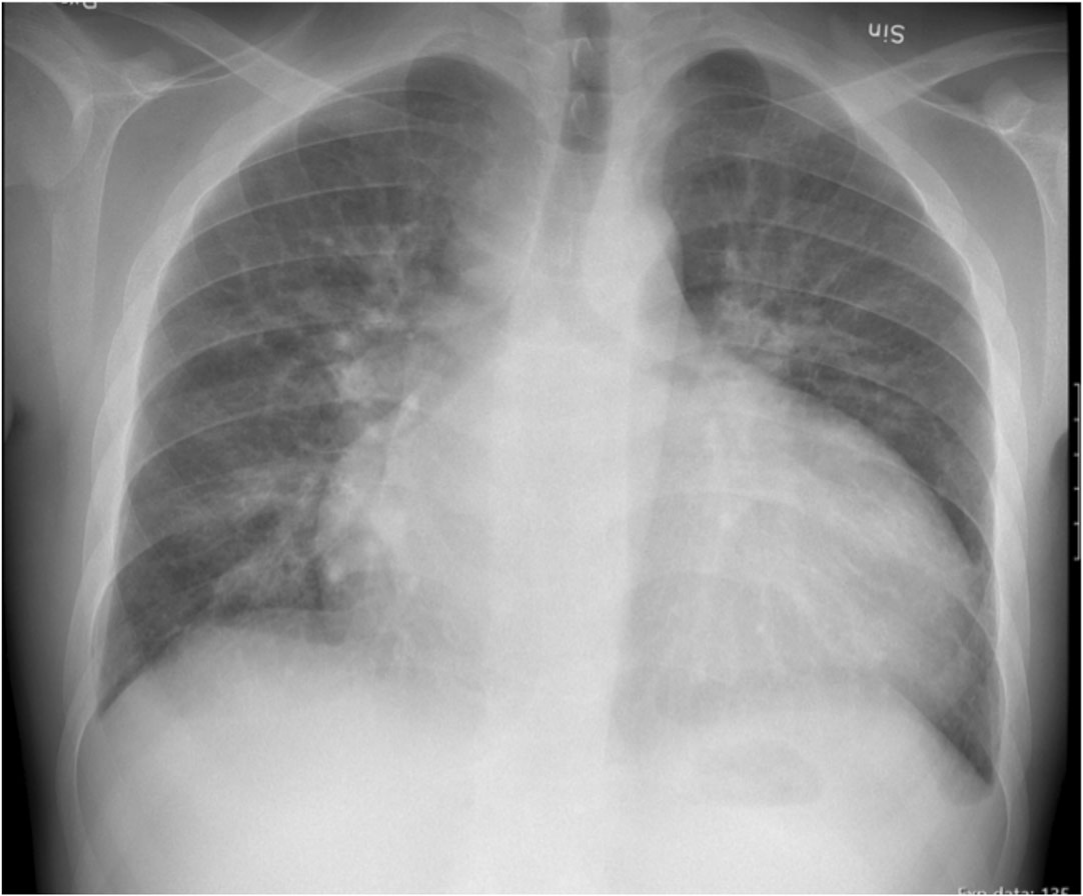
X-ray of the chest after HD visualizing a dilated heart
